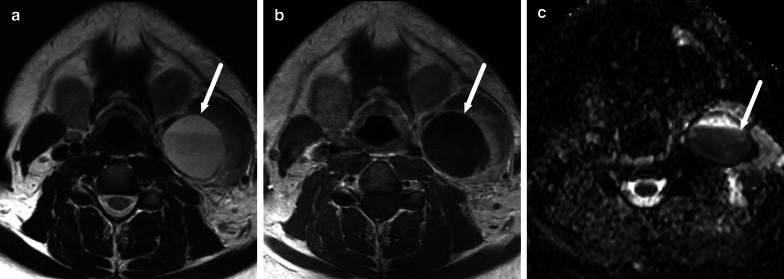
Infected second branchial cleft cyst (pathology proven). MRI shows a large cystic mass (arrows) under a swollen sternocleidomastoid muscle on axial T2-weighted (a) and post-contrast T1-weighted (b) images. ADC map shows a layering of purulent fluid (c), consistent with a secondary infection of a cystic mass
